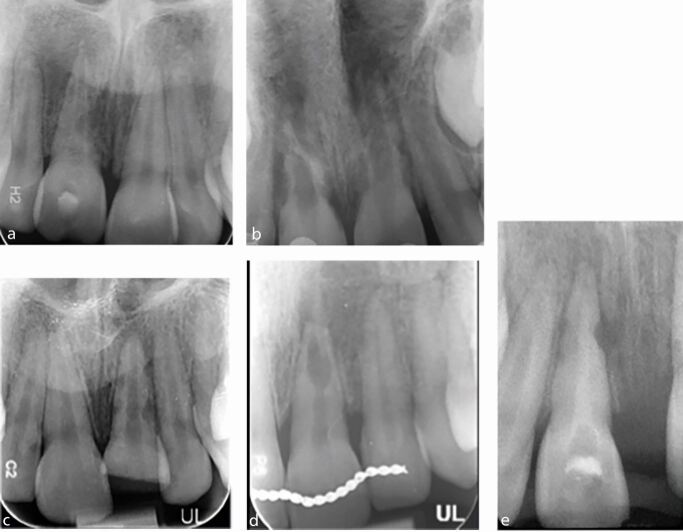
a, b, c, d, e) Series of various periapical radiographs of traumatised teeth experiencing infection-related and replacement-related resorption
